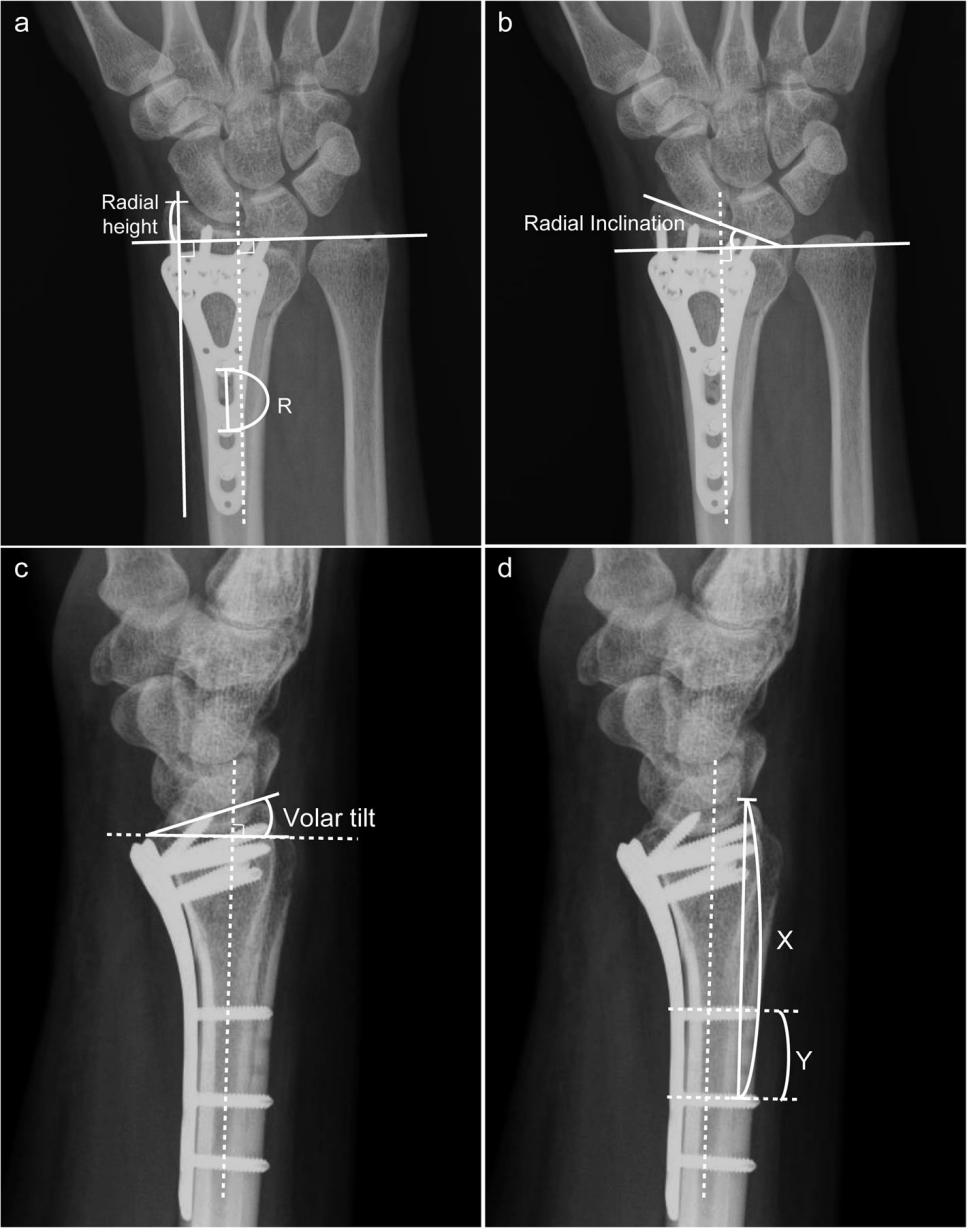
Radiographic measurements. a Radial height measurement. R is the distance from the distal 1st locking hole to the distal 2nd locking hole in the AP view, which should be fixed in each radiograph, so that that the Radial height/R ratio after correction is shown to minimize statistical error. b Radial inclination measurement is as shown in the figure. c Volar tilt measurement is as shown in the figure. d The status of dorsal collapse. X is the distance from the tip of the dorsal cortex to the 2nd distal locking screw in the lateral view. Y is the distance from the distal 1st to the distal 2nd locking screw in the lateral view. Y is a fixed value in each image, so that the ratio X/Y is shown to minimize statistical error
